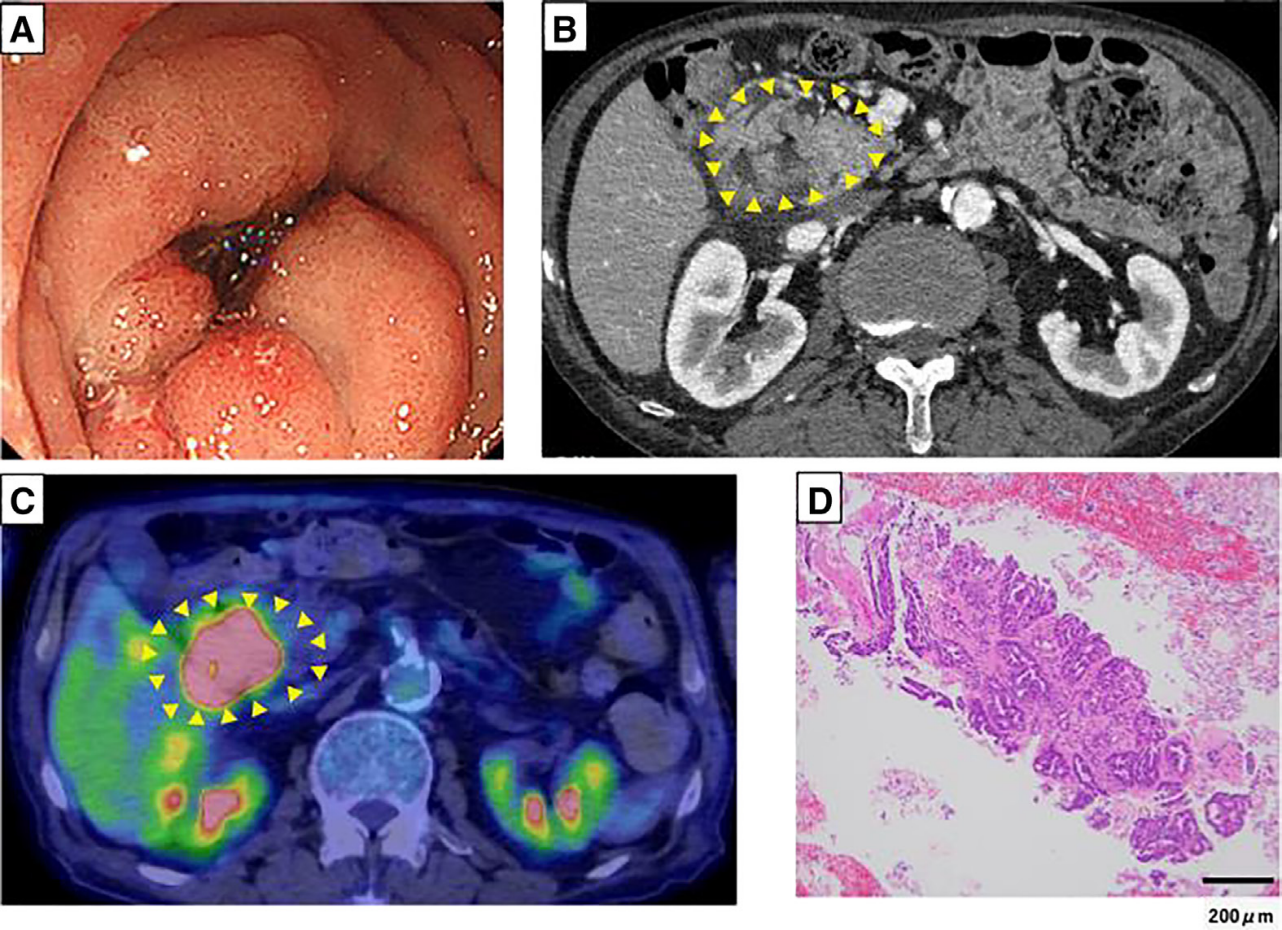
Esophagogastroduodenoscopy revealed circumferential thickening from the supraduodenal ampulla to the descending duodenum, obstructing passage (A). CT showed thickening with discontinuous wall, microperforation, and abscess (arrowheads) (B). PET-CT demonstrated uptake in the lesion (arrowheads) (SUV_max_ 19.6) (C). Biopsy confirmed adenocarcinoma (D; scale bar: 200 μm). SUV_max_, maximum standard uptake value
